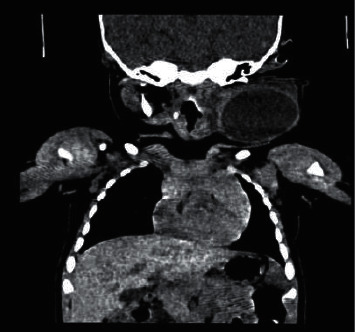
Coronal computed tomography showing left cervical cystic hygroma.
